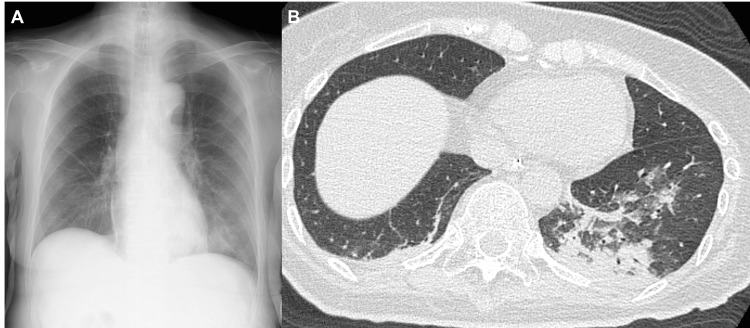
Chest imaging findings on admission (day 1) (A) Chest X-ray Posteroanterior chest radiograph showing consolidation in the left lower lung field. (B) Computed tomography chest CT scan demonstrates consolidation and ground-glass opacities in the left lower lobe. The distribution pattern is consistent with aspiration pneumonia. No pleural effusion or mediastinal lymphadenopathy is observed. These findings led to the diagnosis of aspiration pneumonia and prompted sputum culture, which subsequently yielded the isolate initially suspected as *Corynebacterium diphtheriae*.
